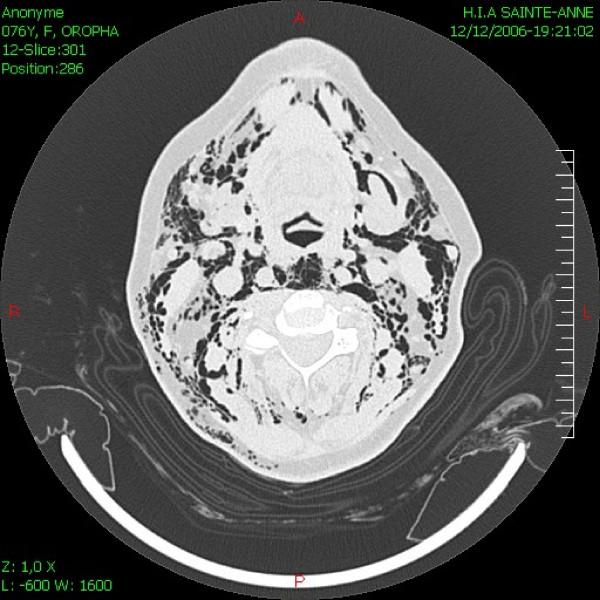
Thoracic CT showing diffuse soft tissues emphysema of the neck.
